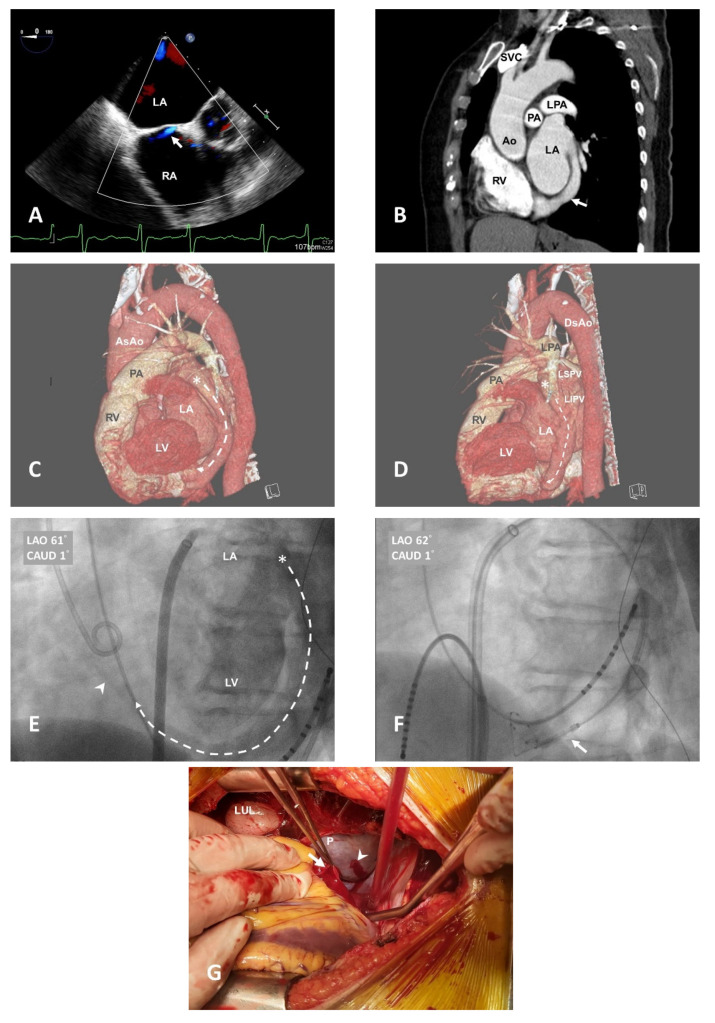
This figure presents the pre-operative images and the intra-operative findings. (A) An ASD-like trivial jet (white arrow) but without obvious defect in the transesophageal echocardiography; (B) An abnormal shunt originating from the LAPFW into the enlarged CS (white arrow); (C) The dashed line indicating the abnormal shunt in the left lateral view of 3D reconstructed CT; (D) The left posterior-lateral view of 3D reconstructed CT indicating that the shunt (dashed line) originated from the LAPFW (*); (E) Left ventriculography (Supplementary Video S1) by trans-septal needle showed the abnormal shunt (dashed line) inflow (*) and the outflow as CS position (arrowhead); (F) The 28 mm cryoballoon (white arrow) was advanced through the shunt for CS ablation (−80 °C, 3 min); (G) by elevating the heart, the ruptured CS (white arrow) and previous pericardiocentesis wound (arrowhead) was exposed intraoperatively. LA: left atrium; RA: right atrium; LV: left ventricle; RV: right ventricle; LAPFW: left atrium posterior free wall; Ao: aorta; PA: pulmonary artery; LPA: left pulmonary artery; SVC: superior vena cava; AsAo: ascending aorta; DsAo: descending aorta; LSPV: left superior pulmonary vein; LIPV: left inferior pulmonary vein; LUL: left upper lobe; P: pericardium.
